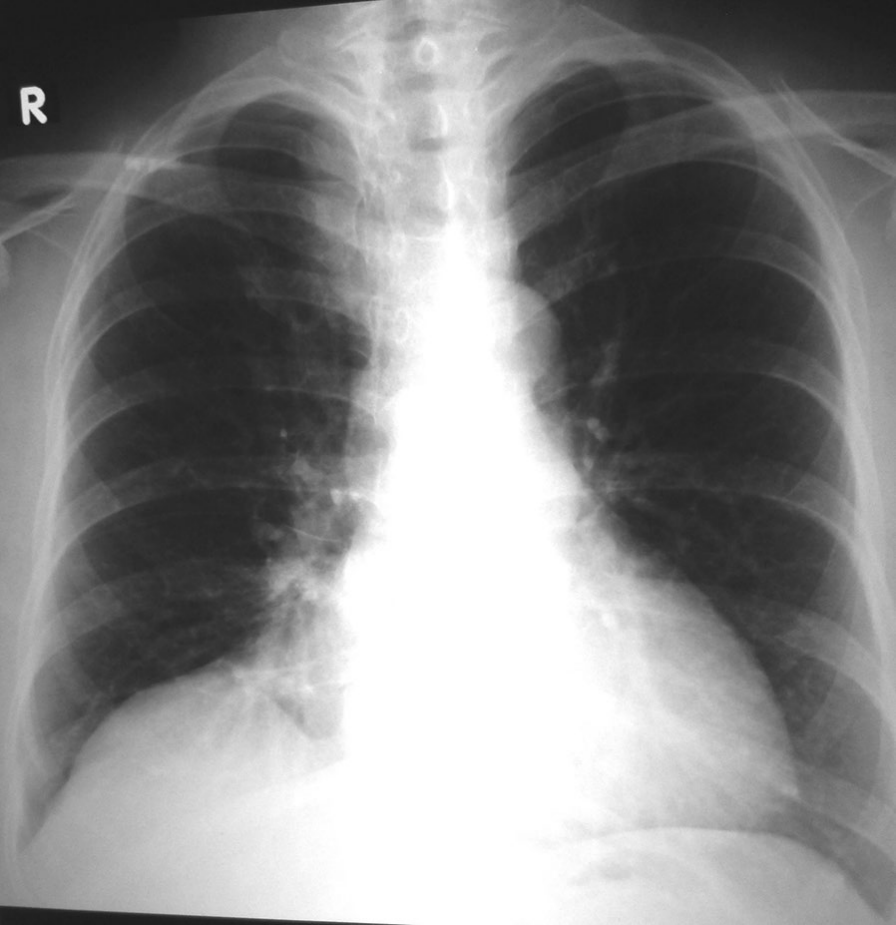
Postoperative chest radiograph showing good result.
